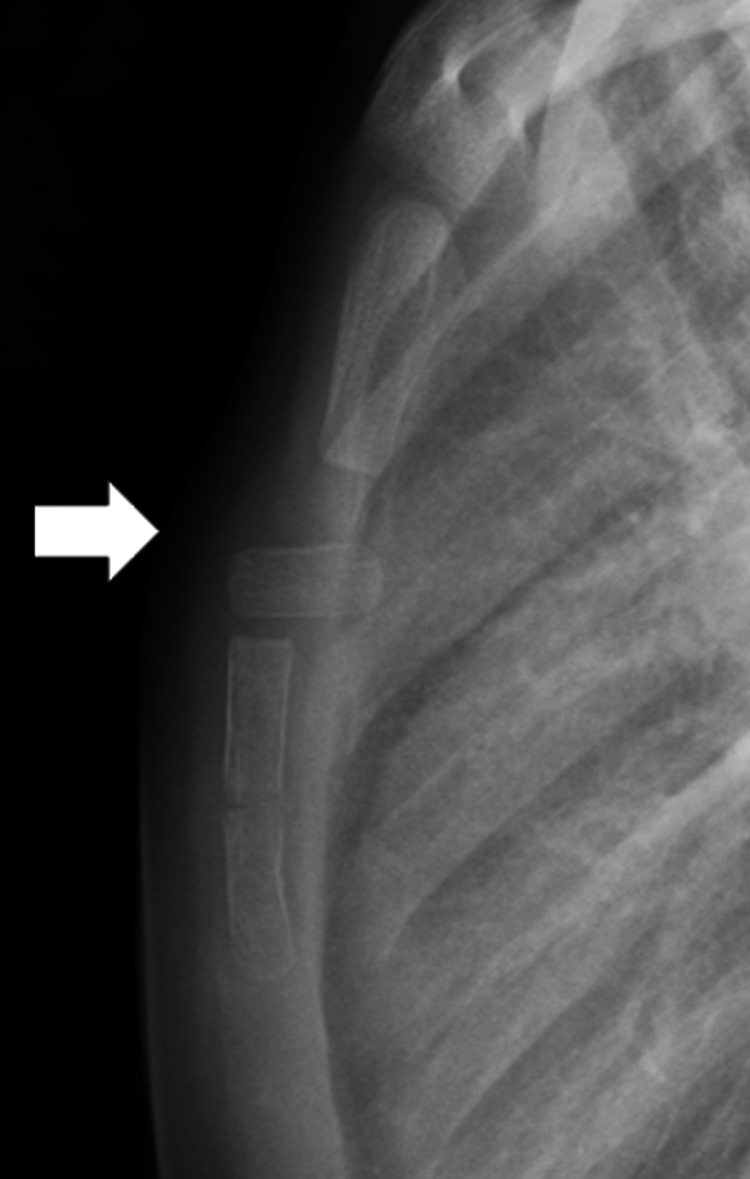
Anterior dislocation of the first sternal segment on radiograph (white arrow).
